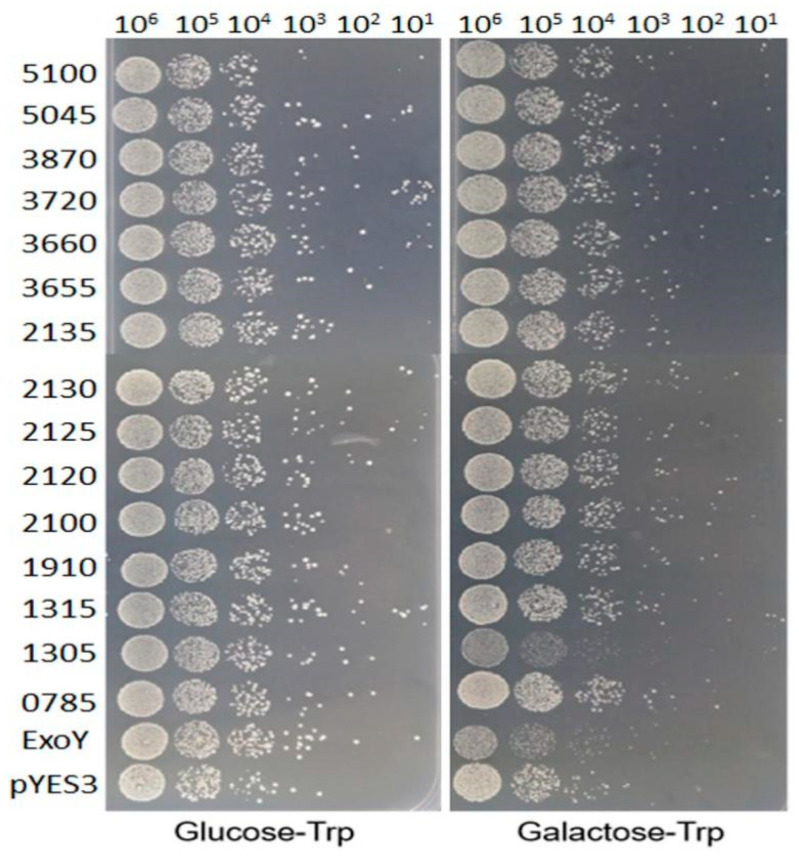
Yeast growth was inhibited by the heterologous expression of a CLas protein FlgI (CLIBASIA_01305). The 15 potential virulence factors of CLas were individually transferred into the yeast expression vector pYES3/CT. Transformed yeast was grown overnight in the repressing medium (2% glucose). Cultures were then normalized to OD_600_ of 1.0, and serial 10-fold dilutions were spotted onto a repressing or inducing solid medium (2% galactose and 1% raffinose). Cell growth was compared to the yeast with the empty vector (pYES) and to the yeast harboring the toxic gene Exoenzyme Y of *P. aeruginosa* (positive control). Photographs were taken after 2 to 3 days of growth at 30 °C. The experiment was performed three times.
